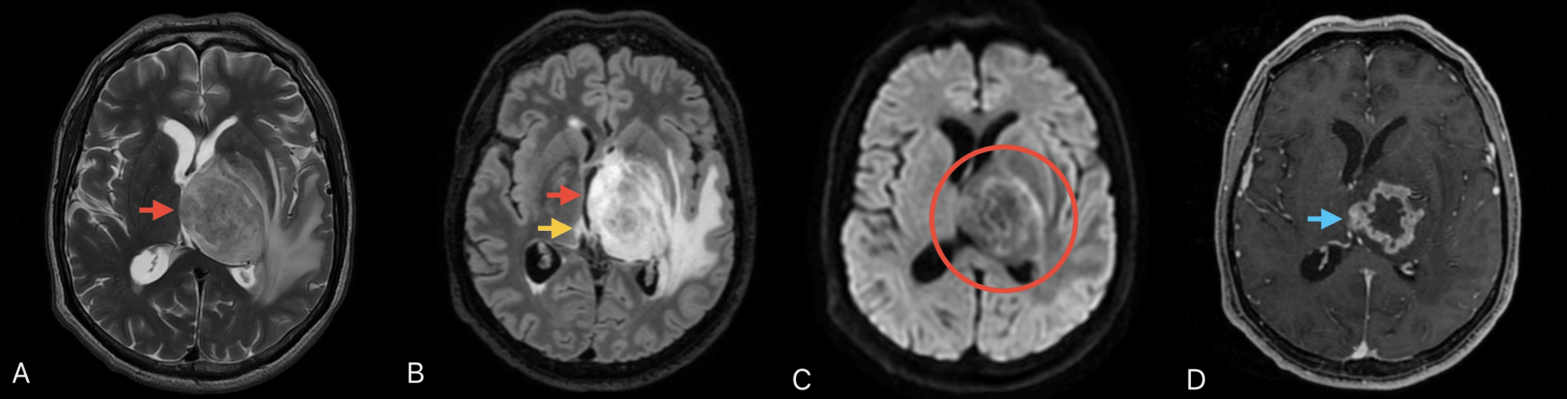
Multisequential axial MRI of the brain showing the left thalamic lesion. On T2 (A) and FLAIR (B) sequences, the lesion (red arrows) appears heterogeneous with mixed high and low signal intensities and surrounding white matter signal changes extending contralaterally through the posterior commissure (yellow arrow). DWI sequence (C) shows areas of high signal intensity (red circle), which were low on the ADC map (not shown), in keeping with diffusion restriction. T1 post-contrast image (D) shows the irregular, thick, "ring" enhancement of the lesion (blue arrow). MRI: magnetic resonance imaging; FLAIR: fluid-attenuated inversion recovery; ADC: apparent diffusion coefficient; DWI: diffusion-weighted imaging
